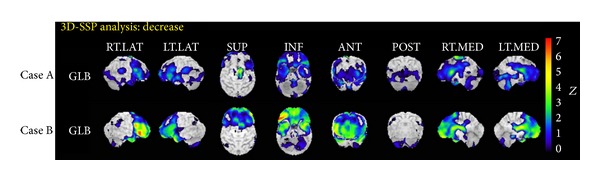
3D-SSP Analysis. Case A. IMP-SPECT images revealed mildly reduced r-CBF in the bilateral frontal lobes of patient 7, who was within normal limits at all of three tests. Case B. IMP-SPECT images revealed moderately reduced r-CBF in the bilateral frontotemporal lobes of patient 22, who failed all three tests.
